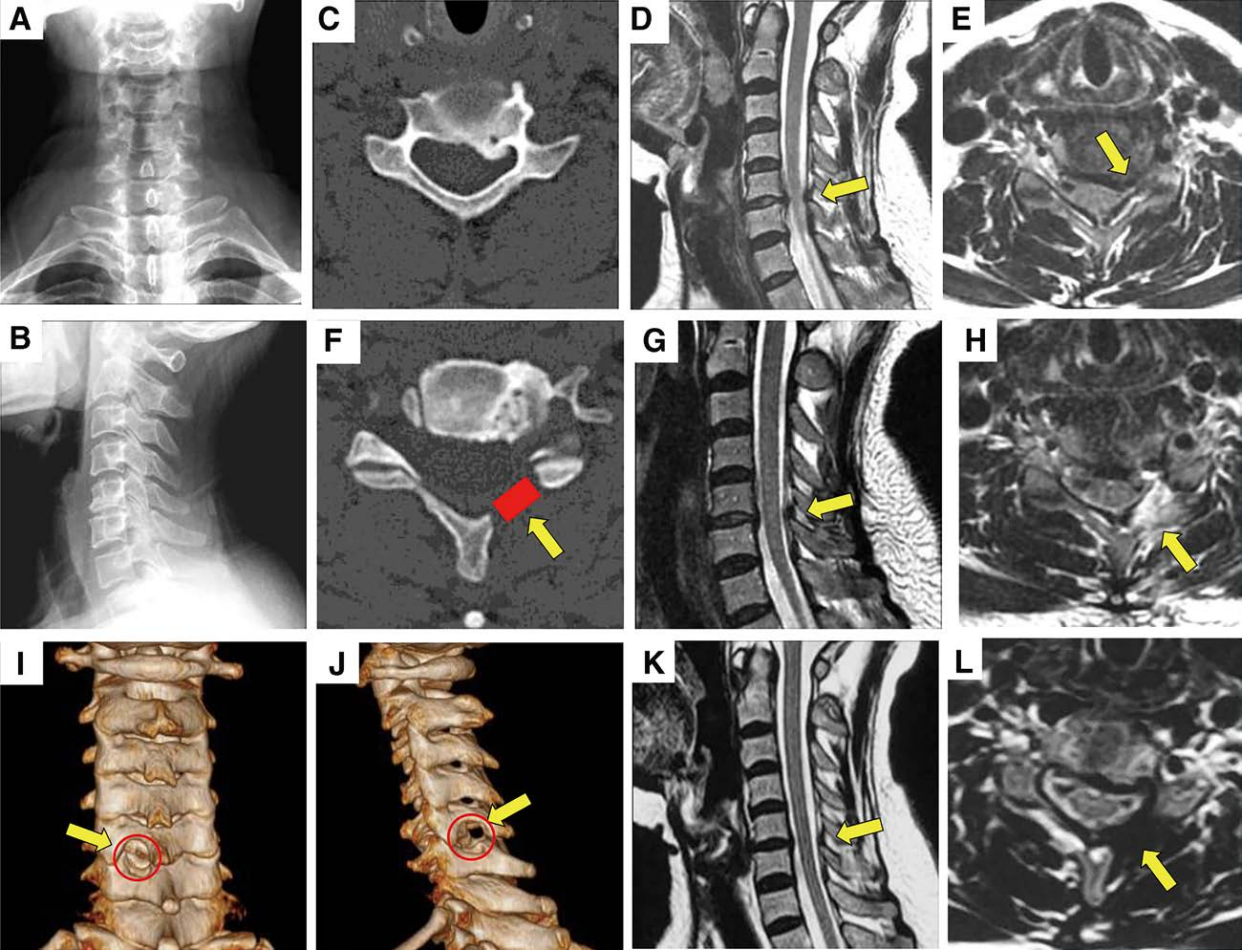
A 48-yr-old female patient with CSR of C5–6 underwent PPECLF. Preoperative radiographic images, including radiographic (A and B), CT (C) MRI (D, E). Postoperative radiographic images, including CT (F), MRI (G and H), CT 3-dimentional reconstruction (I and J). The red zone indicates the range of dorsal decompression. Two year after surgery, the decompression remained unchanged (K and L). CSR = cervical spondylotic radiculopathy, CT = computed tomography, MRI = magnetic resonance imaging, PPECLF = posterior percutaneous endoscopic cervical laminoforaminotomy.
